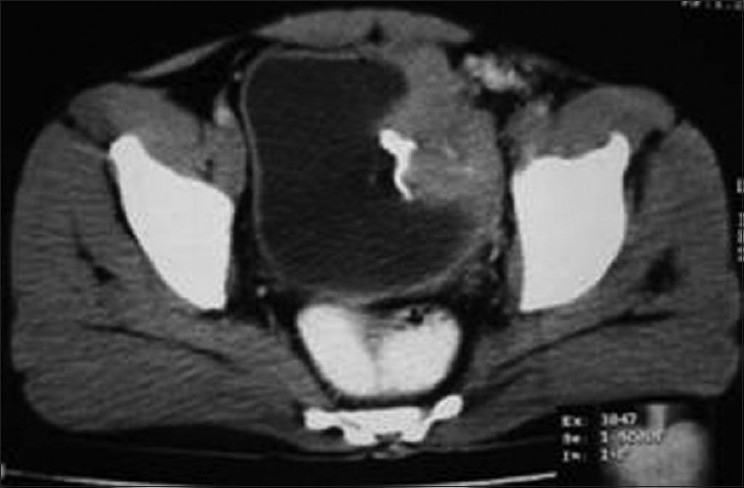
Computed tomography of the pelvis shows lobulated mass with contrast enhancement on left lateral wall of bladder
